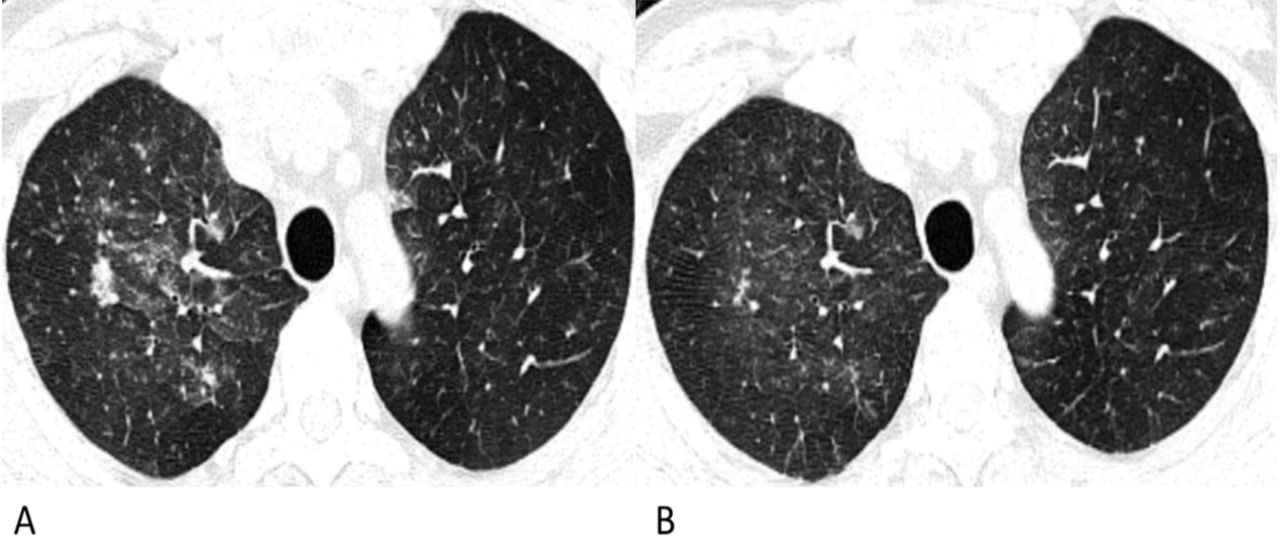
Follow-up chest CT images of lymphoma-associated pneumonia. A 49-year-old man was diagnosed with diffuse large B-cell lymphoma, and received chemotherapy with rituximab before the chest CT. He had a fever during the chemotherapy, but had no epidemiological history of COVID-19. (A) On the initial chest CT, diffuse patchy GGOs with several solid nodules are shown in both upper lobes. (B) After antibiotic and symptomatic treatment, the lesions in the follow-up CT are diminished, and the density decrease.
